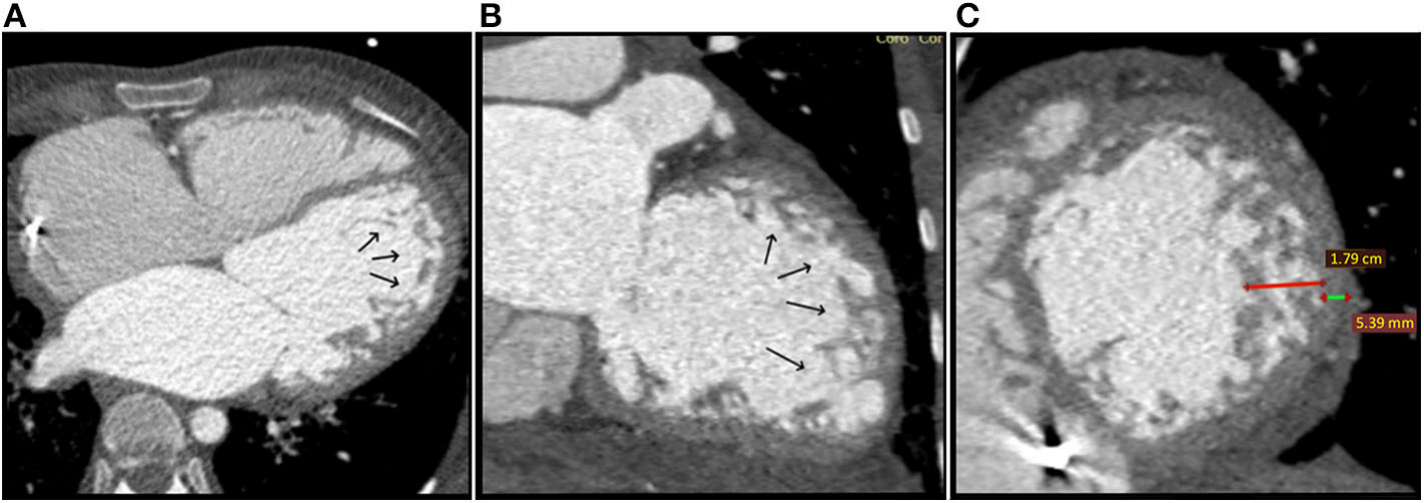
Case 2: Multi-detector Computed Tomography (MDCT) angiography at the moment of diagosis: (A) Axial reconstruction and (B) Coronal reconstruction: Black arrows show prominent trabeculations and deep intertrabecular recesses located at the apex and lateral wall of the LV; (C) Sagital reconstruction, short axis plane showing non-compaction (red line) to compaction (green line) ratio of > 3.3 (17,9/5,39 mm).
